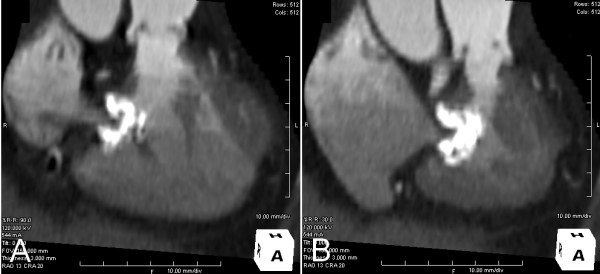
Multislice cardiac enhanced CT: systolic RVOT narrowing is observed. (A) Diastole. (B) Systole.
